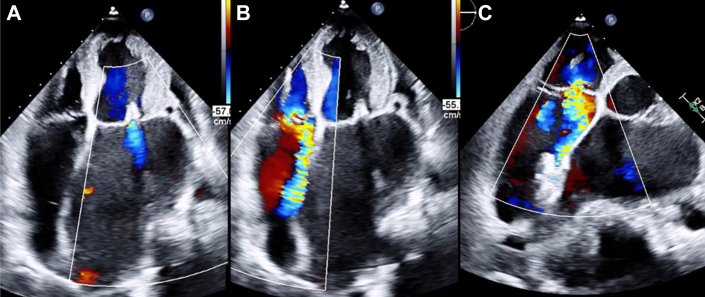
Postprocedural Recovery and Device Stability Transthoracic echocardiography on postprocedural day 1. (A) Stable mitral repair (mild mitral regurgitation) and elimination of the iatrogenic shunt. (B) Reduction in TR to a moderate level. (C) The persistent TR jet is seen directly impinging on the occluder, which acts as a mechanical barrier, protecting the friable septum from further tearing. TR = tricuspid regurgitation.
